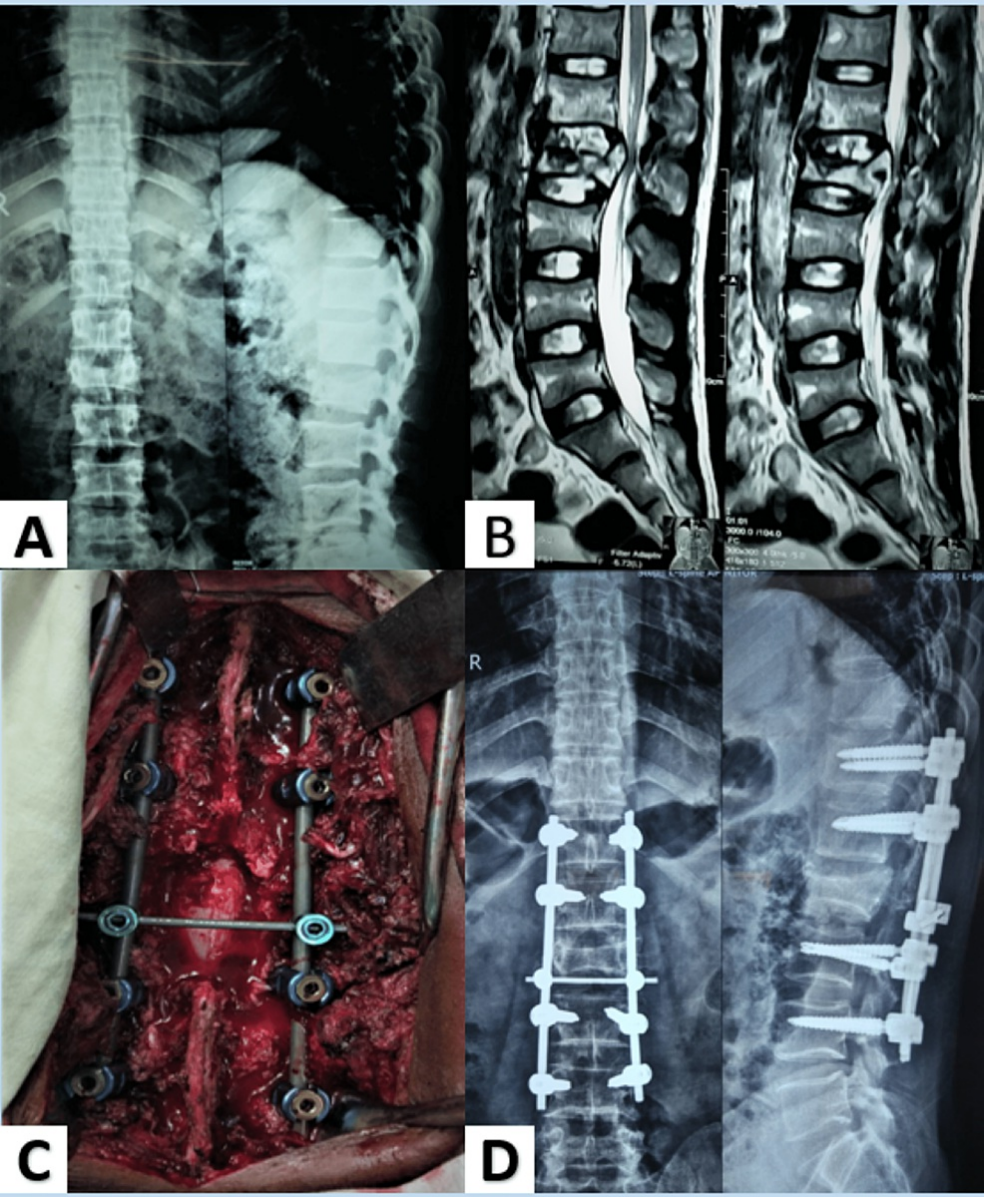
Long-segment posterior fixation. A. Preoperative X-ray of the lumbosacral spine anteroposterior and lateral view. B. T2-weighted sagittal section (MRI of the lumbosacral spine) showing burst # L2. C. Peroperative image showing long-segment pedicle fixation with cross-link. D. X-ray of the lumbosacral spine anteroposterior and lateral view at the final follow-up.
